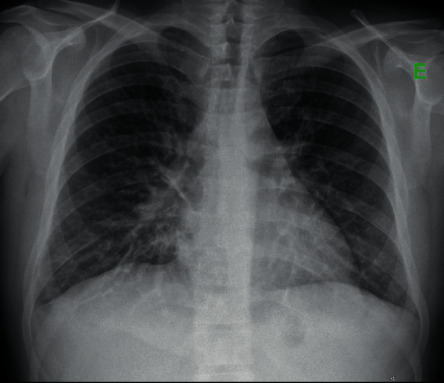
Chest X-ray performed on the 2^nd^ trip to the ER: bilateral perihilar reinforcement, erasure of both costophrenic sinuses, and diffuse interstitial infiltrate are observed.
